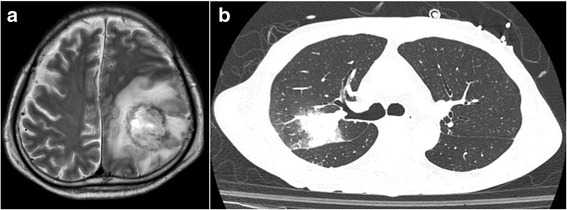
Image inspection findings. a T2-weighted magnetic resonance imaging scan of head. b Computed tomography scan of lung
